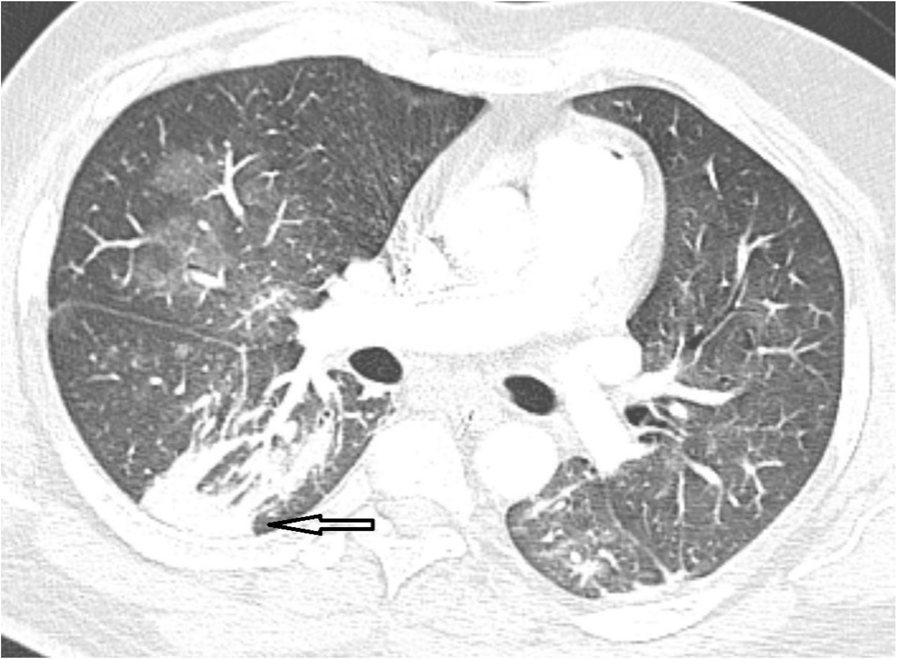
CT scan of COVID-19 patient shows Multiple areas of GGO as well as reticular pattern at anterior segment of upper lobes as well as superior segments of lower lobes
